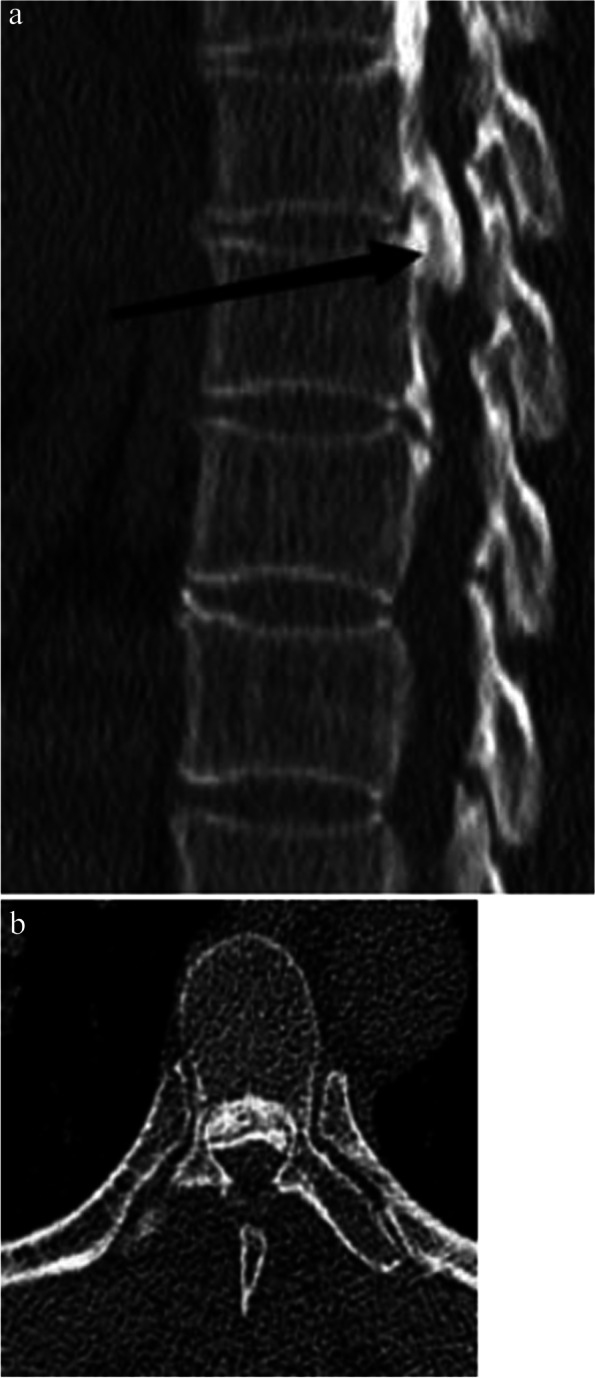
a sagittal bone window CT demonstrating the double-layer sign in T-OPLL. b axial bone window CT demonstrating the double-layer sign in T-OPLL
